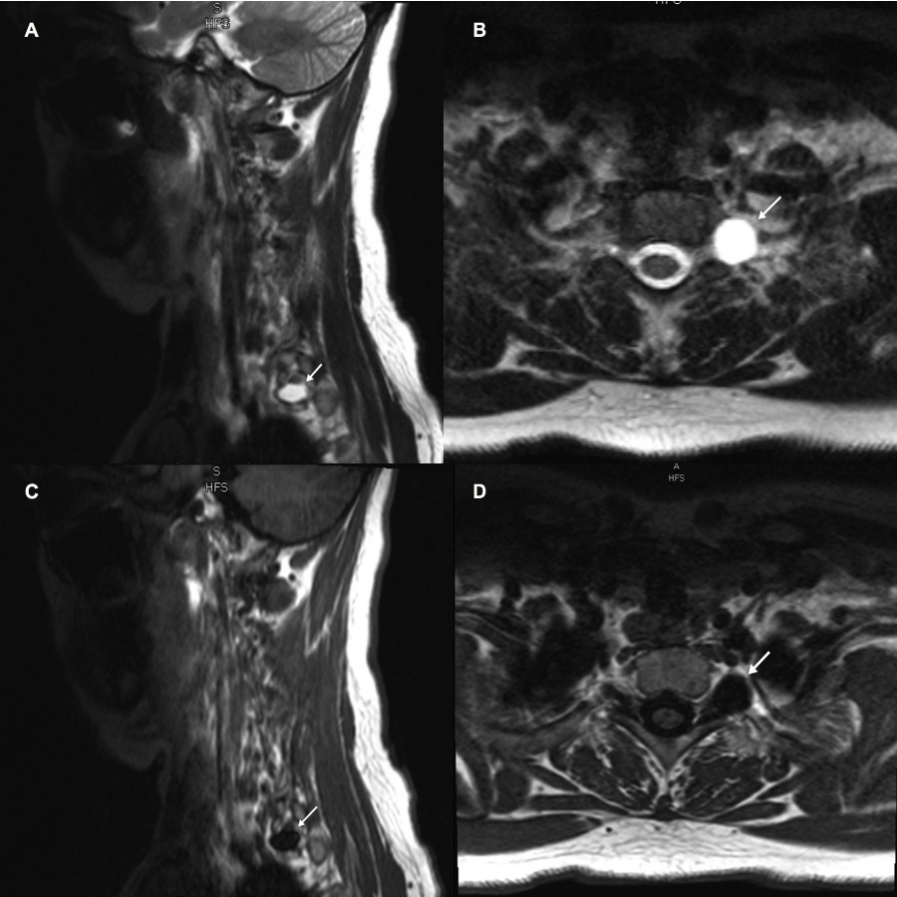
MRI of cervical spine without contrast [A] T2 weighted sagittal images show a hyperintense lesion (white arrow) that originates from the T1 nerve root and extends to the T1-T2 left neuroforamen; [B] T2 weighted axial images show a hyperintense lesion (white arrow) that originates from the T1 nerve root and extends to the T1-T2 left neuroforamen; [C] T1 weighted sagittal images show a hypointense lesion (white arrow) that originates from the T1 nerve root and extends to the T1-T2 left neuroforamen; [D] T1 weighted axial images show a hypointense lesion (white arrow) that originates from the T1 nerve root and extends to the T1-T2 left neuroforamen.
